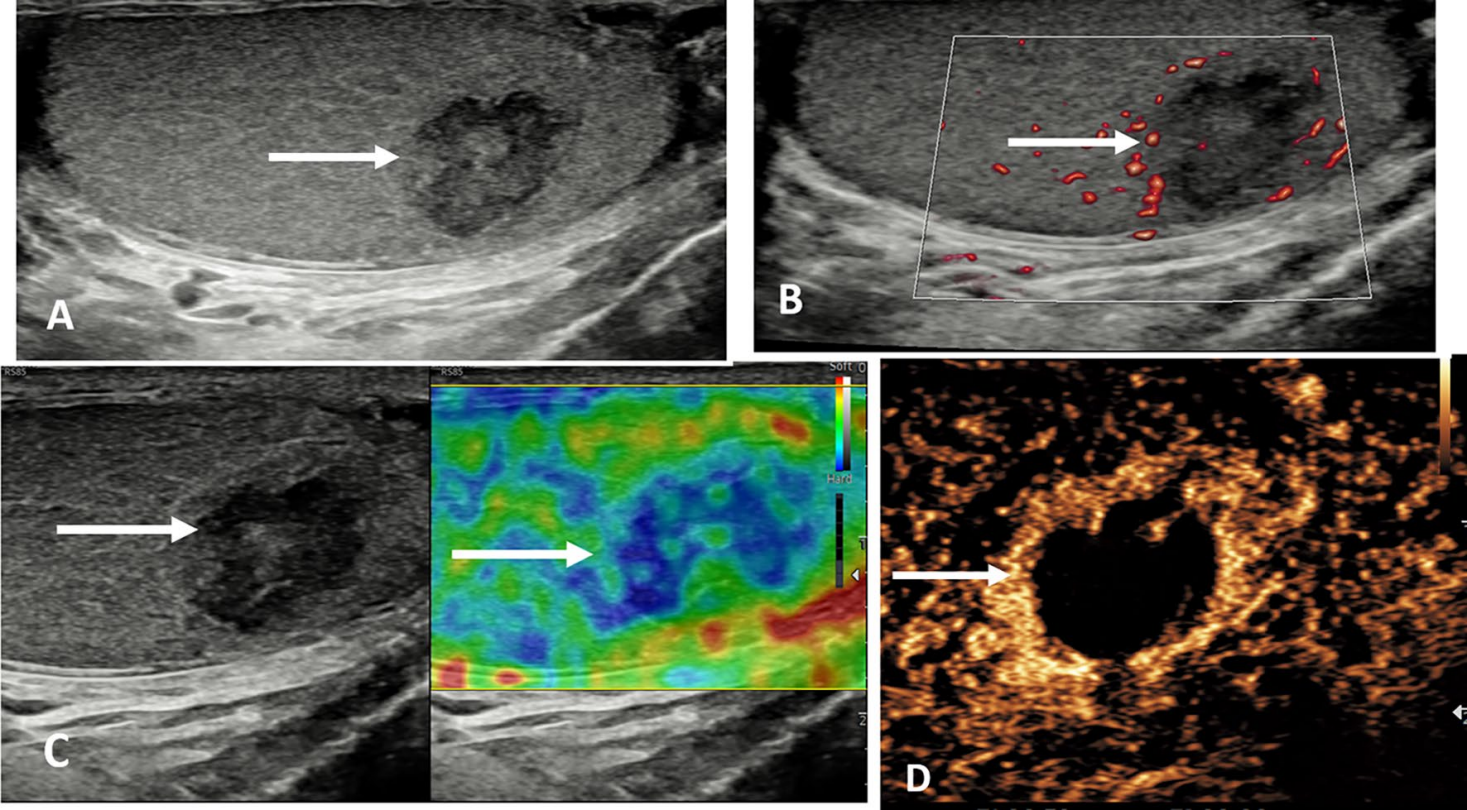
Presentation with acute testicular discomfort in a 62 year old; segmental testicular infarction. A B-mode longitudinal view of the left testis demonstrating a irregular focal lesion in the lower aspect of the testis (arrow). B Microvascular imaging shows color surrounding the lesion with possible internal faint color Doppler flow (arrow). C Tissue elastography demonstrates a mixed stiffness lesion, predominantly of increased stiffness (arrow); this will evolve over time. D The contrast enhanced ultrasound examination confirms an avascular lesion with surrounding hyperemia (arrow)
